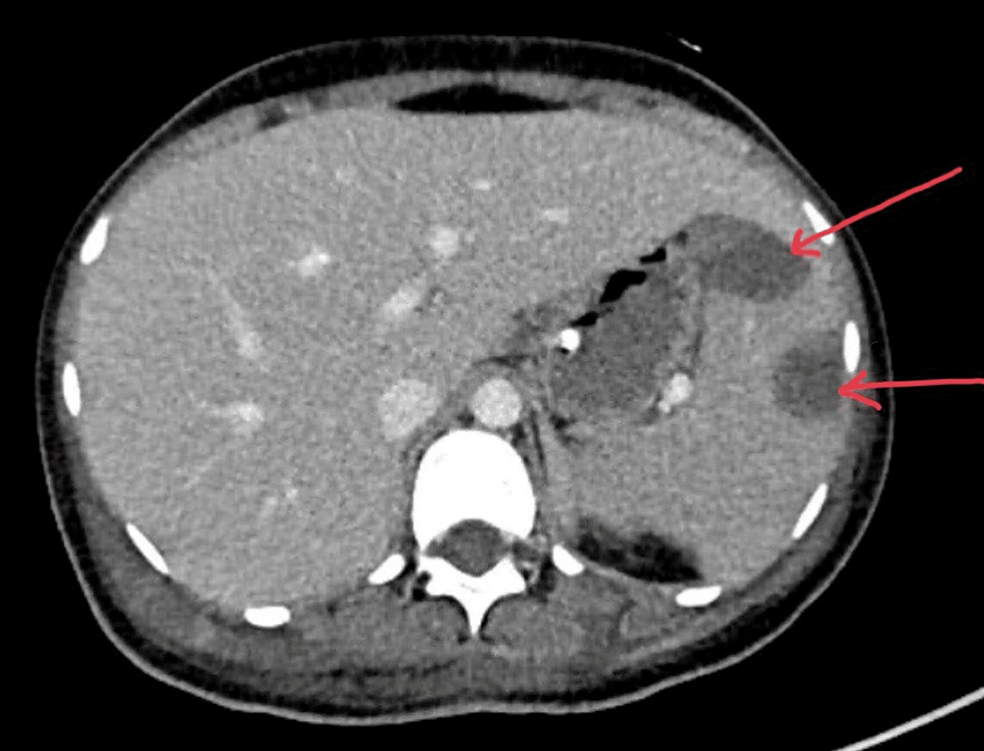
Axial view of CT abdomen shows two large wedge-shaped hypodense lesions representing infarcts.
